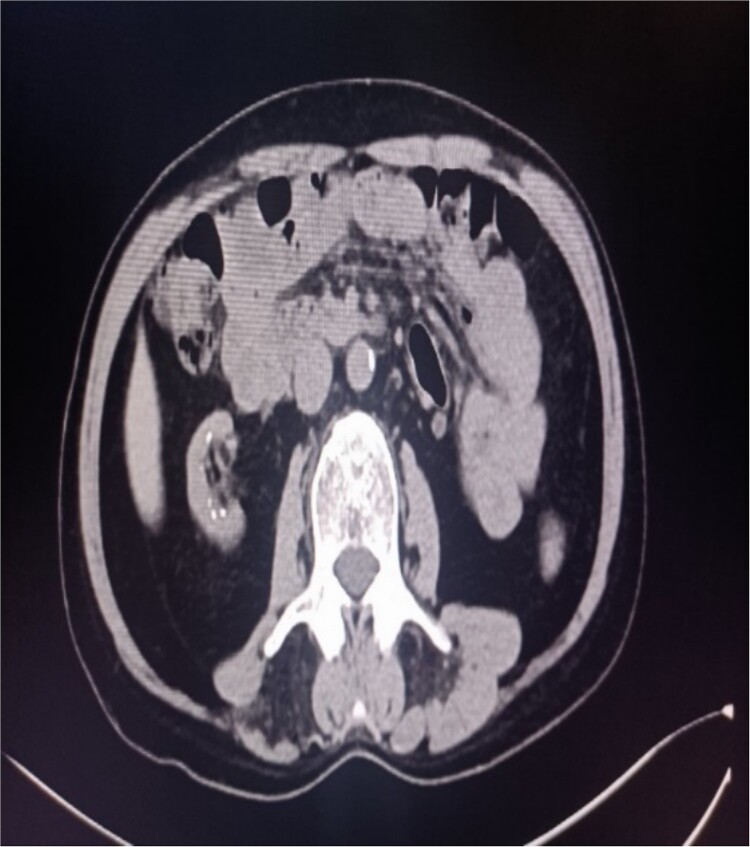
Abdominal CT showing multiple punctate stones in the right renal area *in situ*.
